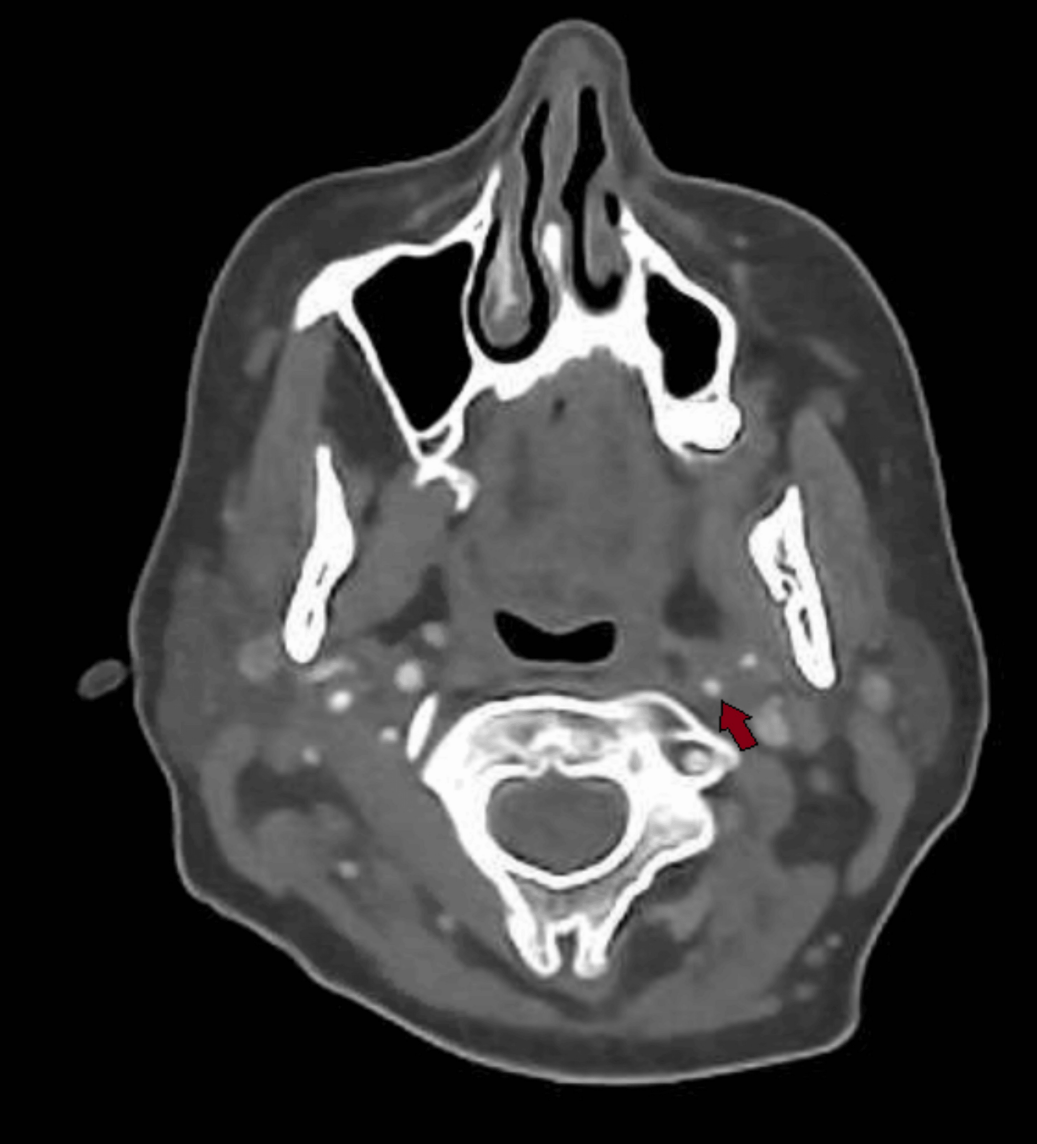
Axial CT carotid angiogram showing the left extracranial internal carotid artery with poor luminal filling seen.
